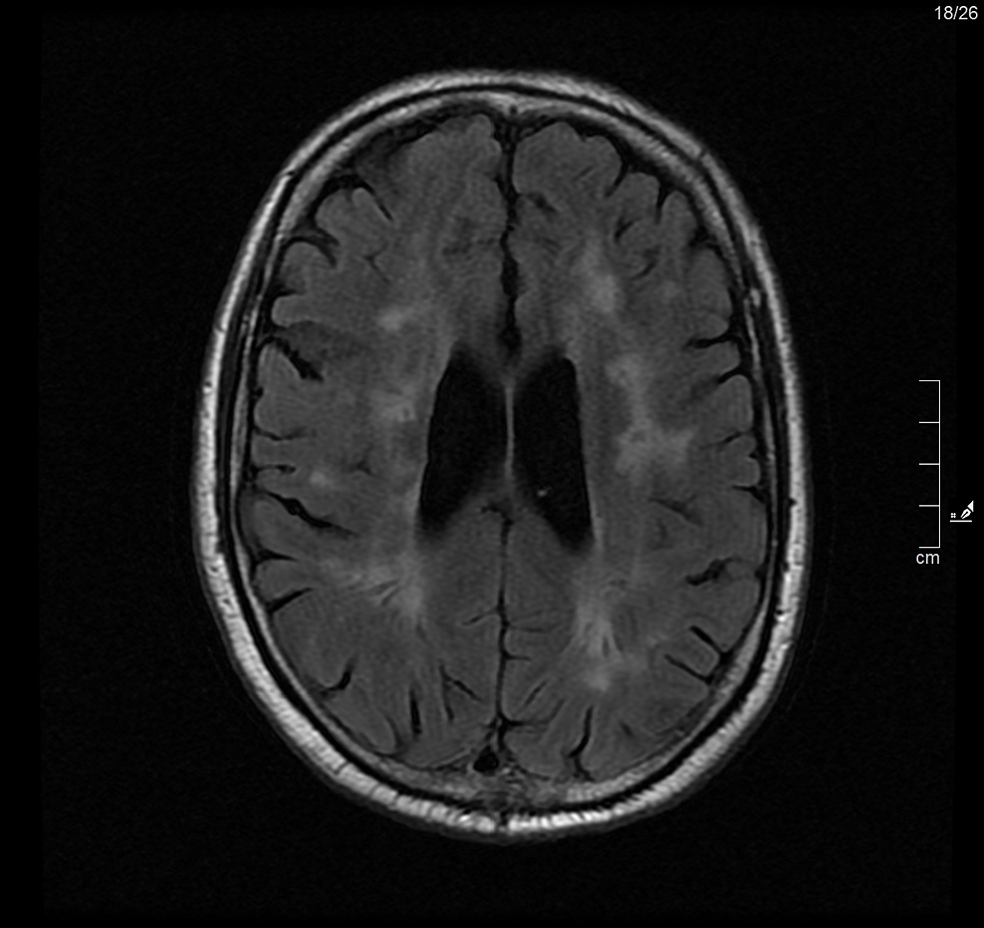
MRI of the brain - FLAIR T2 sequence - diffuse changes in the periventricular and deep subcortical white matter Change in signal intensity with hyperintense areas and lesions in FLAIR T2 sequence located in periventricular white matter, radiated crowns and subcortical white matter of both cerebral hemispheres.
